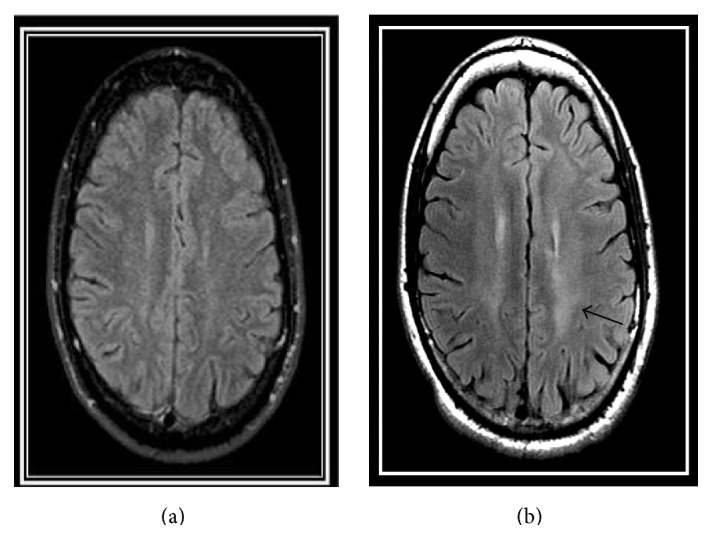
MRI-FLAIR protocol. Left: initial MRI, 04/12. Right: MRI following relapse 10/12. Note a diffuse hyperintense, ill-defined, left hemispheric centrum semiovale lesion superior to the lateral ventricles (black arrow).
